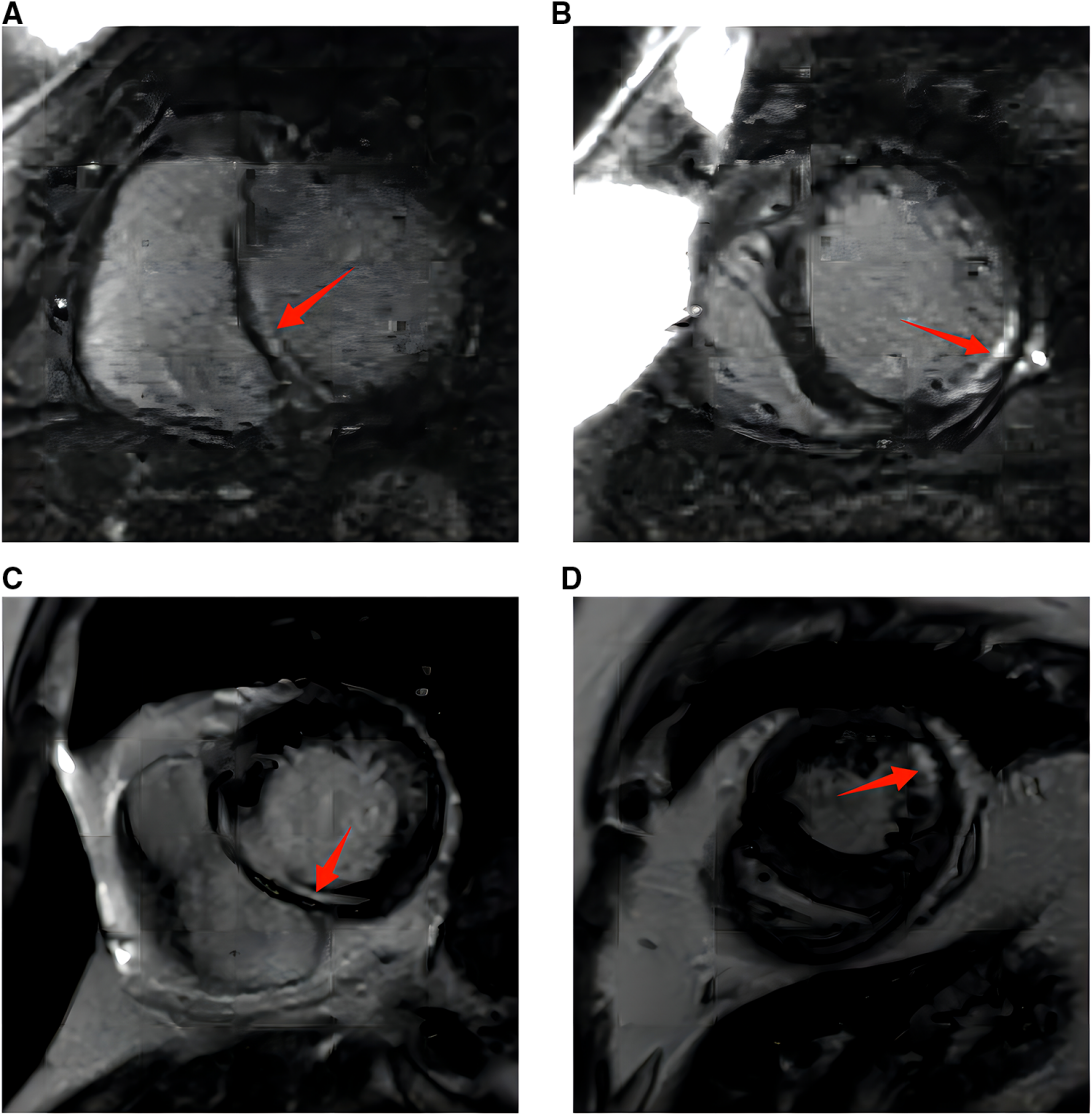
(A) Cardiac magnetic resonance imaging with gadolinium at diagnosis. The red arrow marks late gadolinium enhancement in the interventricular septum (bright liner enhancement). (B) Cardiac magnetic resonance imaging with gadolinium at diagnosis. The red arrow marks late gadolinium enhancement in the inferior and lateral walls of the left ventricle [or left ventricular something (bright liner enhancement)]. (C) Cardiac magnetic resonance imaging with gadolinium at 10 months. The red arrow marks late gadolinium enhancement in the interventricular septum (bright liner enhancement). (D) Cardiac magnetic resonance imaging with gadolinium at 10 months. The red arrow marks late gadolinium enhancement in the inferior and lateral walls of the left ventricle (bright liner enhancement).
